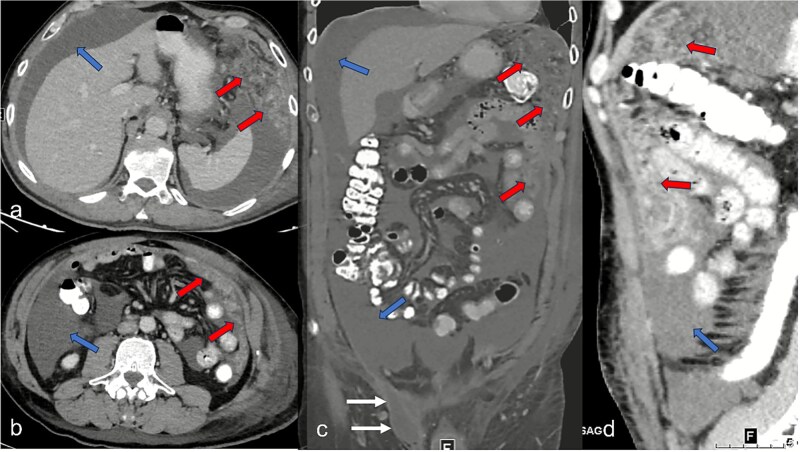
Abdominal CT imaging shows clear ascites (blue arrows) in all quadrants in the axial segments (a and b), the coronary segment (c), and sagittal segment (d), and a tumor-specific structure with thickening of the peritoneum and the greater omentum (red arrows). In (c) representation of the hernial orifice (white arrows).
